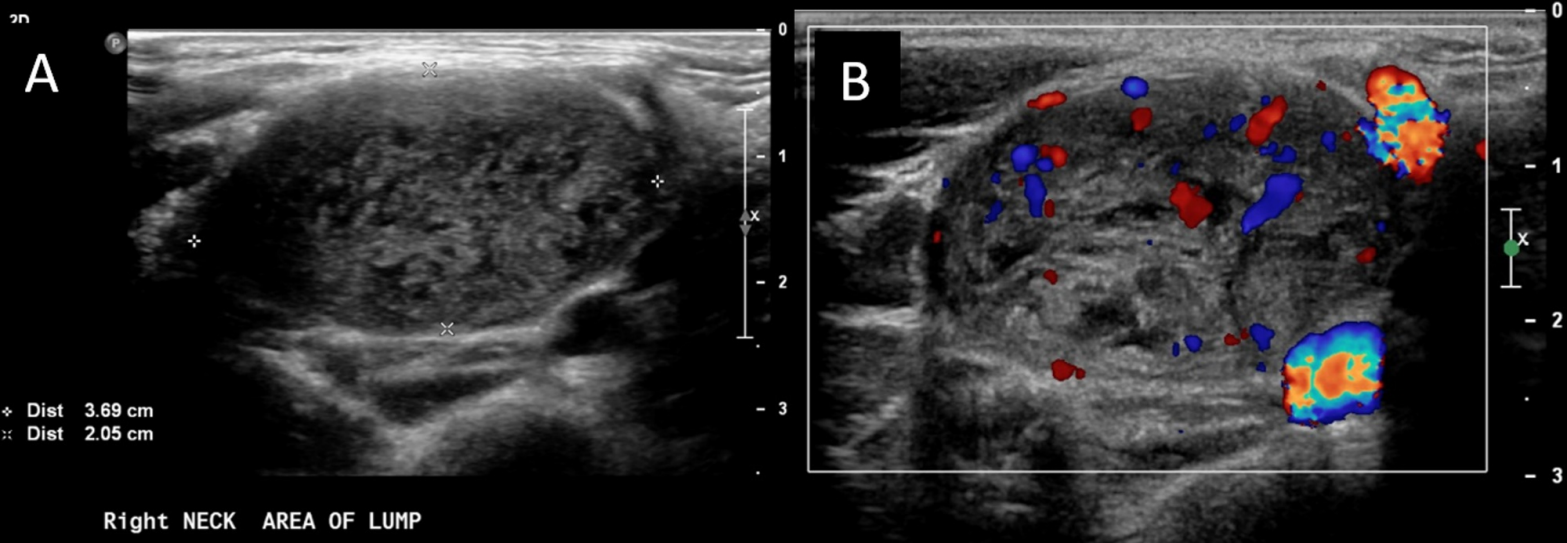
A: Longitudinal ultrasound showing a heterogeneous ovoid mass. B: Internal hypervascularity as demonstrated by Doppler.
